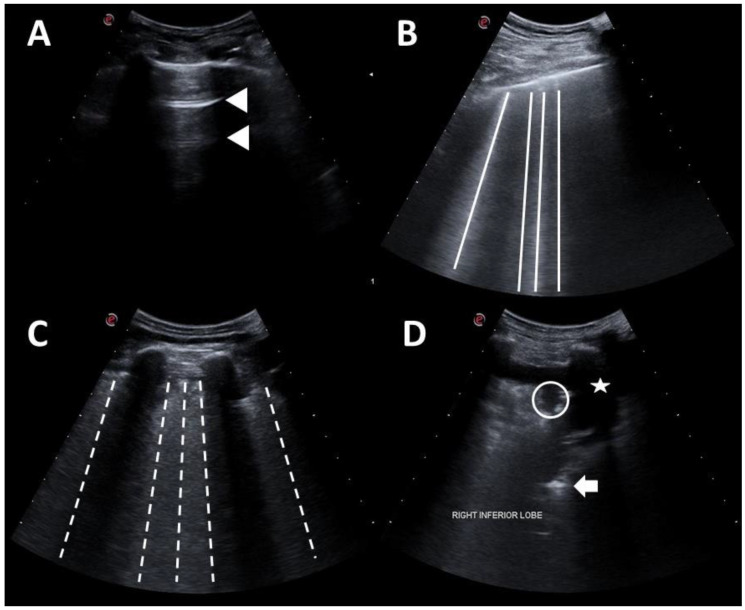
Lung ultrasonography grading score is part of BLUE protocol for evaluation of dyspnoea in emergency setting. (A) Score 0: normal pattern, A-lines or <3 B-lines; (B) score 1: moderate loss, ≥3 B-lines; (C) score 2: severe loss, coalescent B-lines; (D) score 3: complete loss, white lung and/or lung consolidations. Legend: pleural line is indicated by star; A-lines are indicated by triangles; B-lines are indicated by continue lines; coalescent B-lines are indicated by dashed lines; Consolidation is indicated by circles, aerial bronchogram is indicated by arrow, while pleural effusion is indicated by stars.
